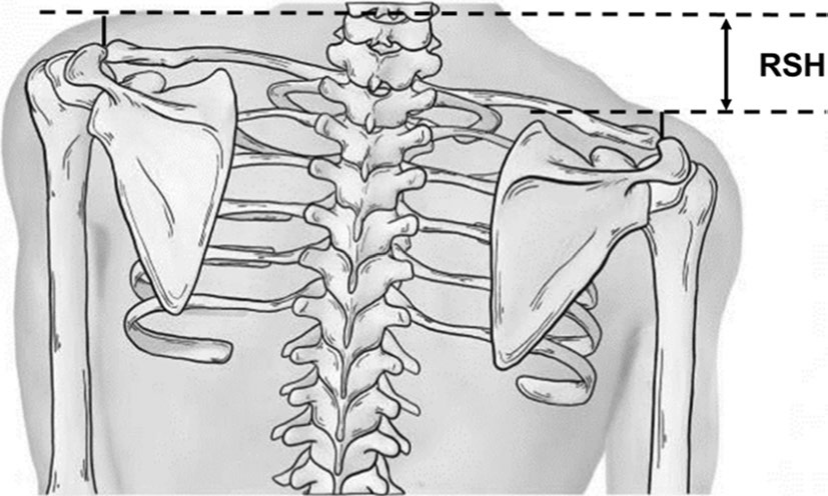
Measurement in the coronal plane: shoulder balance, defined by radiographic shoulder height (RSH)
